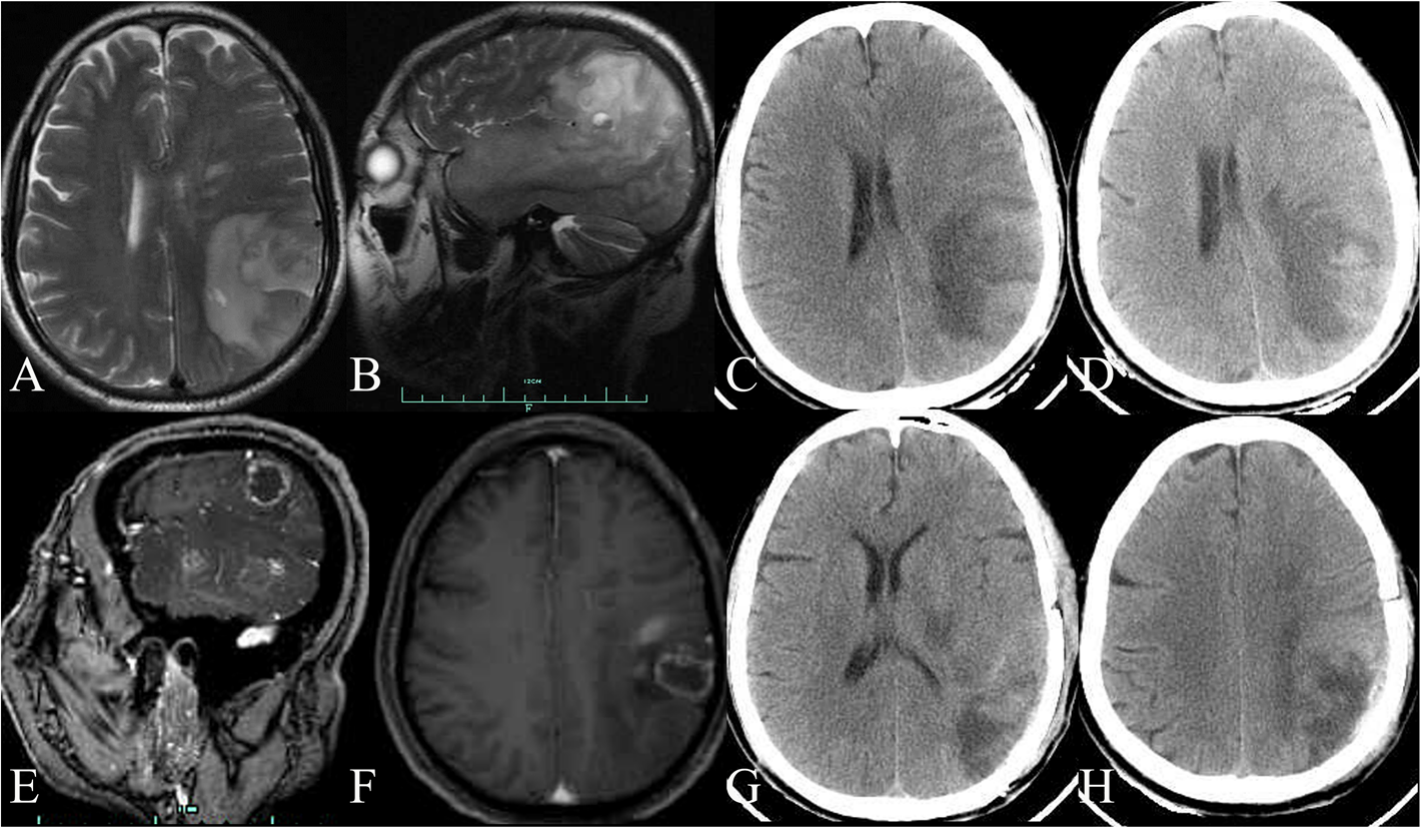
Images 2 months after carotid artery stenting. a, b (2015-3-24): T2-weighted image showed a massive lesion with edema in the left temporal and occipital lobes. c (2015-3-24): CT image also found a massive lesion with edema in the left temporal and occipital lobes. d (2015-3-29): The repeat cranial CT revealed that cerebral edema worsened. e, f (2015-4-11): contrast T1-weighted images showed multiple ring-enhanced lesions with massive edema in the left temporal and occipital lobes. g, h (2015-4-29): Repeat cranial CT scans showed edema regressed 2 weeks post-operatively
